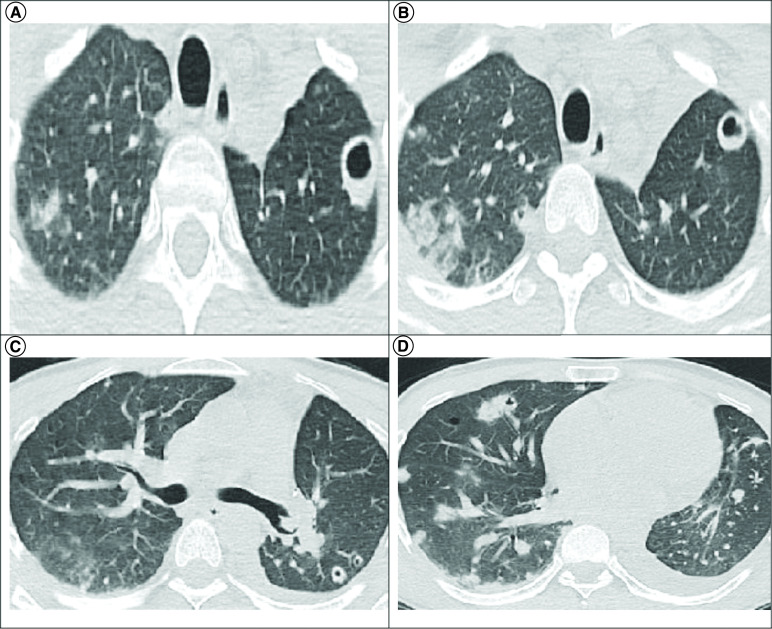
Chest computed tomography images of the patient.
